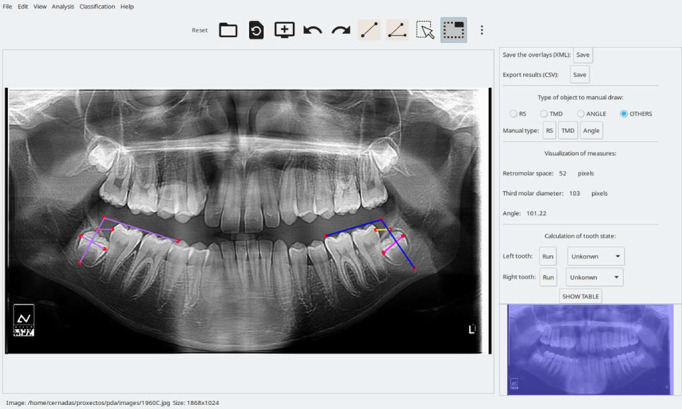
Screenshot of the PDApp software with the panoramic radiography analysis and lateral panel opened. In the left molar, the pink line represents the third molar diameter, the yellow line represents the retromolar space, and the blue lines represent the angle. The measures of the right molar are shown in the lateral panel.
